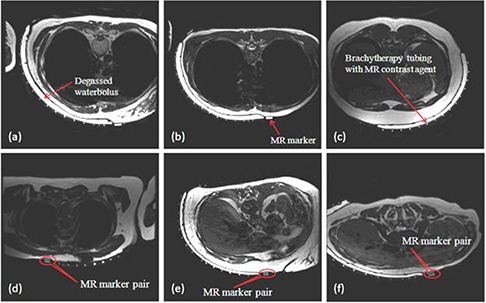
MR images of volunteer CW: (a)–(c) axial images of mastectomy and healthy volunteers with excellent conformity of TBSA to CW; MR images indicate MR visible marker pairs on the skin and TBSA that were used to assess applicator lateral displacement. The bright dots above the water bolus from the liquid MR contrast filled inside the parallel array of brachytherapy catheter channels were used to assess radial displacement of HDR catheters from the CW surface.
